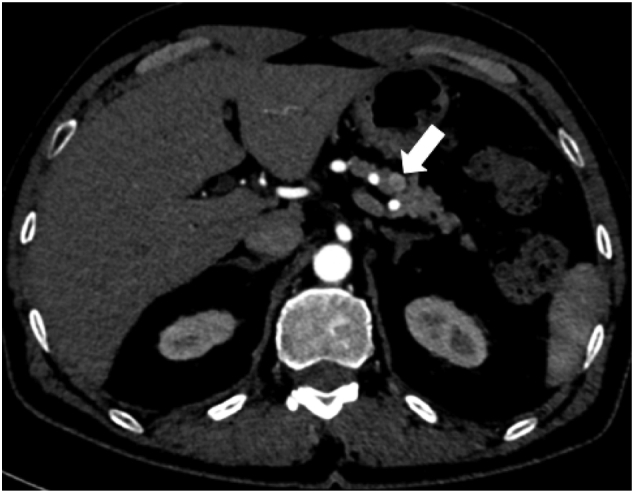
Preoperative contrast-enhanced computed tomography. A hypervascular tumor that was 10 mm in diameter in the pancreatic body was revealed.
